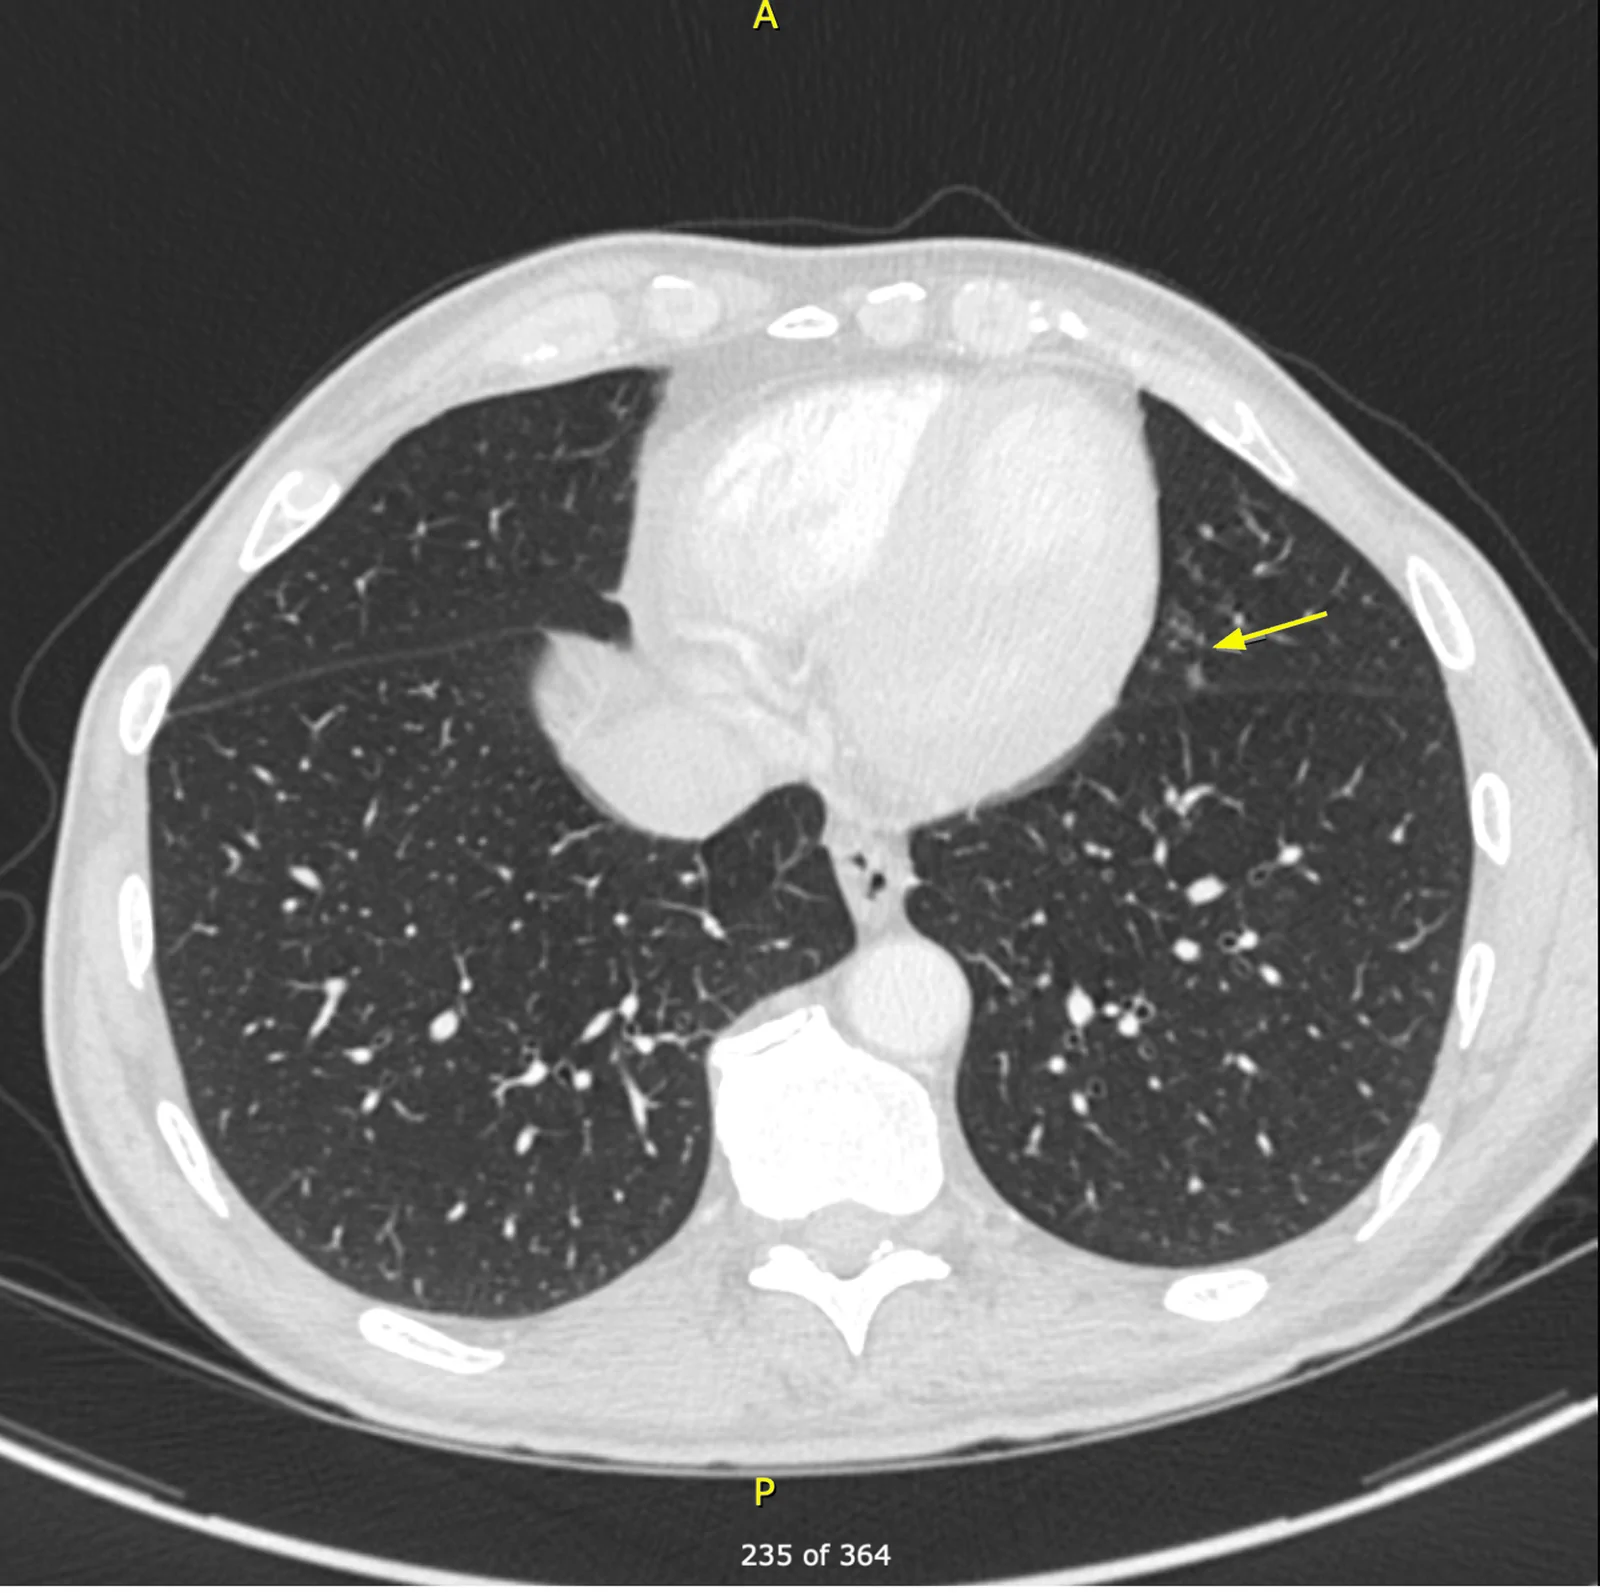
Axial CT chest demonstrating few clustered centrilobular solid micronodules in the inferior lingula (yellow arrow), without evidence of metastasis disease elsewhere in the chest.
